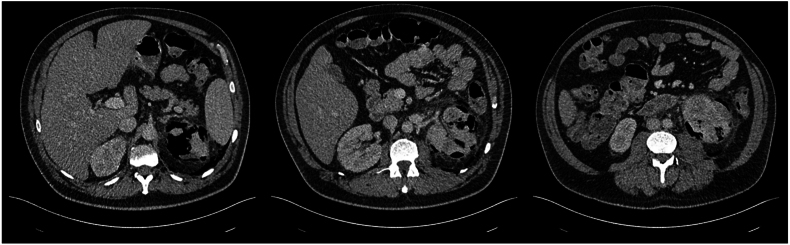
CT Abdomen and Pelvis (CTAP) on admission demonstrating Class IIIb Emphysematous Pyelonephritis with retroperitoneal and para-renal gas extension.
